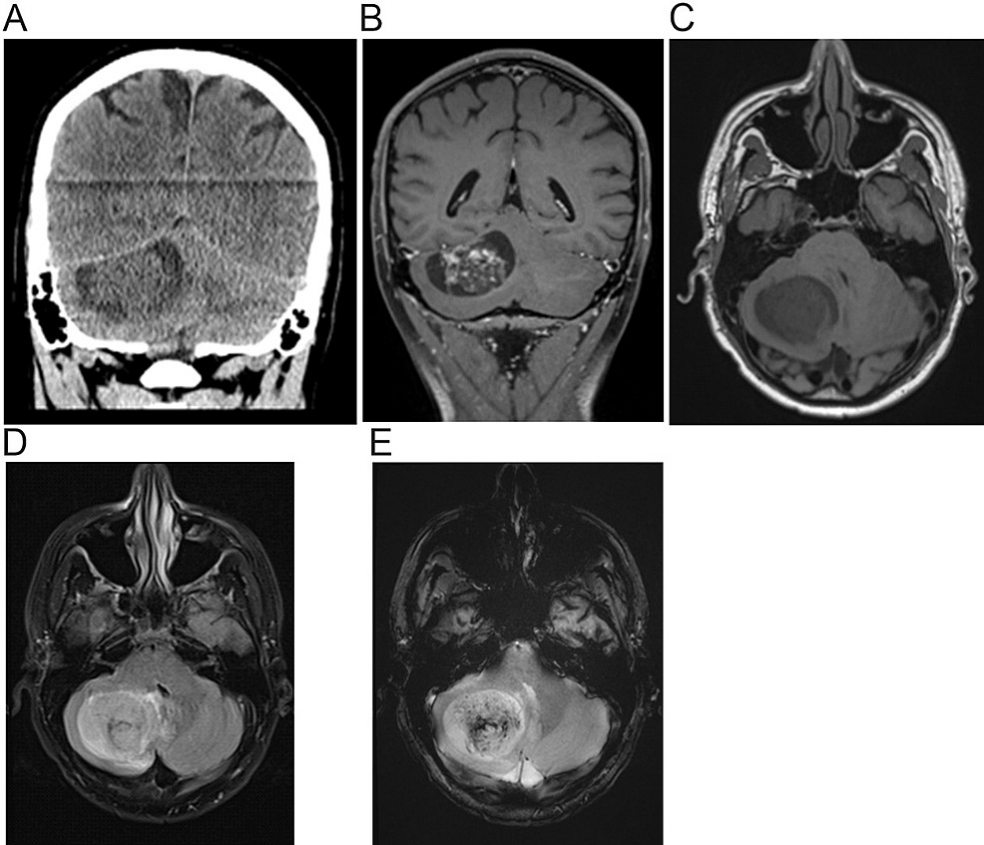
CT scan and MRI of the brain prior to craniotomy (A) CT scan of the head without contrast showing a 42 x 48 x 32 mm round right cerebellar mass with regional mass effect and effacement of the right paramedian cistern. A hemorrhage within the center of the mass is noted. Partial obliteration of the fourth ventricle without appreciable dilatation of the third and lateral ventricles as well as mild descent of the right cerebellar tonsil by about 3.5 mm. (B-E) MRI [(B): T1 (longitudinal relaxation time) with contrast, (C): T1 without contrast, (D): Fluid-attenuated inversion recovery (FLAIR), and (E): Gradient echo sequences (GRE)] of the brain showing a 49 x 4 x 37 mm cystic, solid enhancing hemorrhagic right cerebellar mass with local mass effect with partial effacement of the fourth ventricle, mass effect on the right posterior brainstem, and mild inferior protrusion of right cerebellar tonsil below the foramen magnum. No hydrocephalus.
